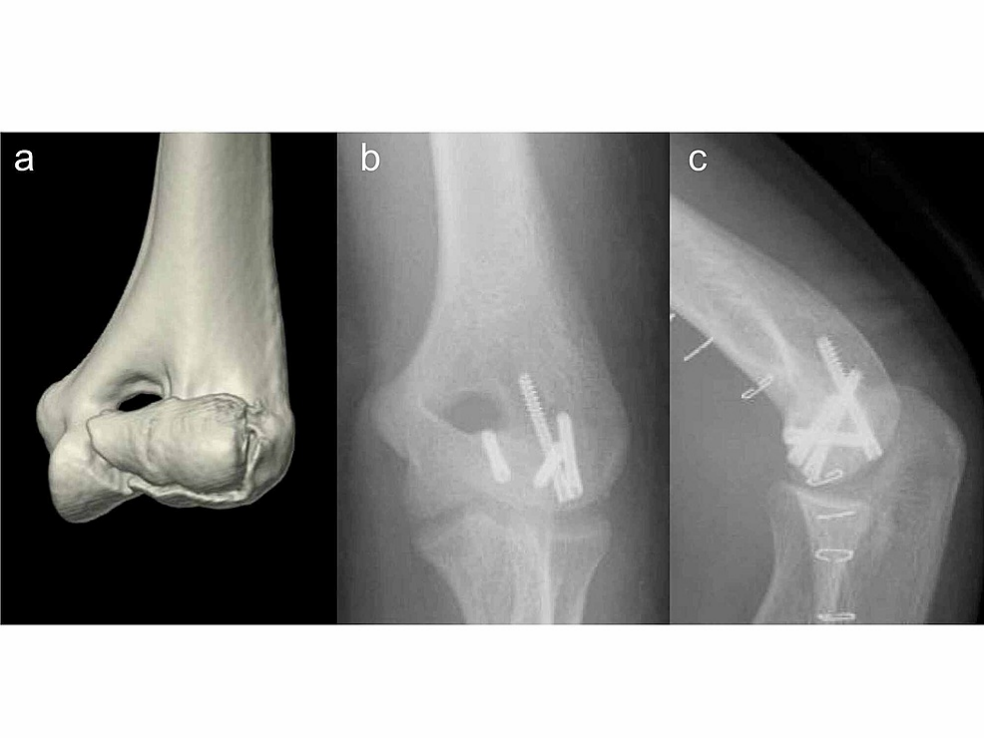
(a) Preoperative CT scan of a 15-year-old male patient with a Dubberley type 2A capitellum fracture. (b) Anteroposterior and (c) lateral X-ray views show the fragment fixation with headless bone screws.
